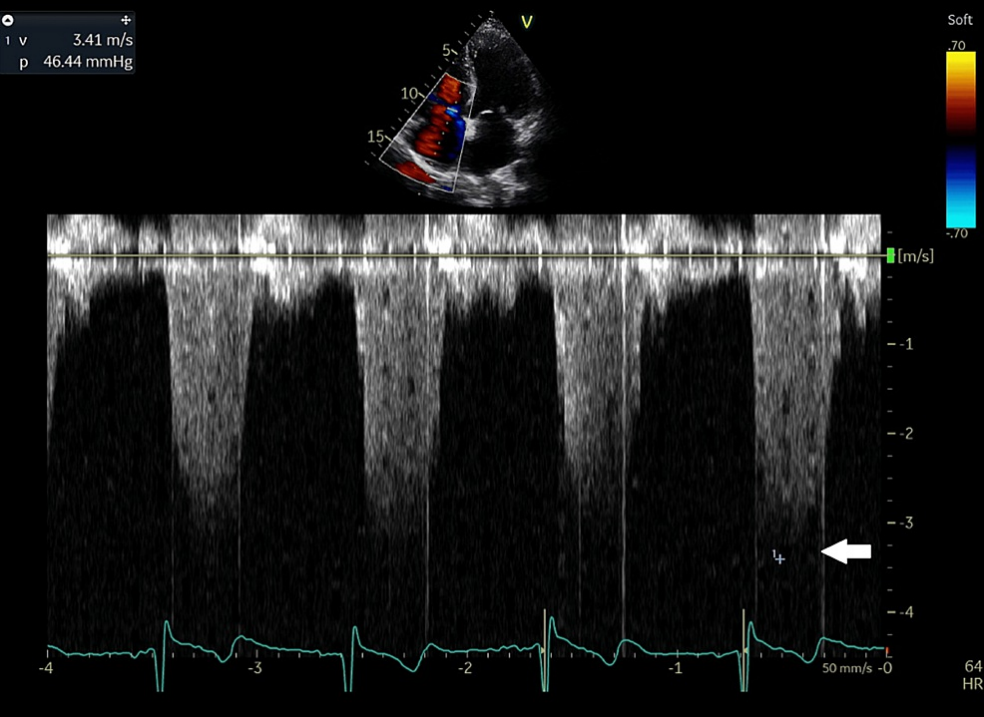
Tricuspid regurgitation jet velocity, recorded by CW Doppler in the apical 4-chamber view, was 3.41 m/s (indicated by a white arrow), allowing for the calculation of systolic pulmonary artery pressure at 51 mmHg. m/sec: meter/second; CW: Continuous wave.
